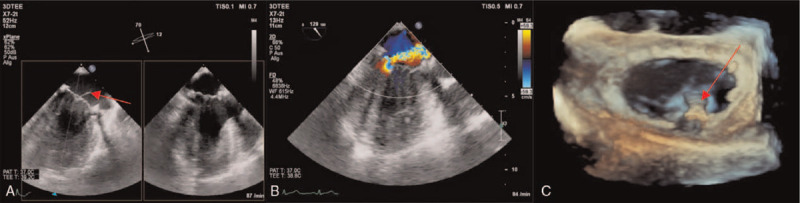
TEE of mitral valve after surgical repair with recurrent regurgitation before intervention. Panel A: Biplane view of the mitral valve. Red arrow indicates a flail leaflet. Panel B: Long axis view of the mitral valve with an anteriorly directed, eccentric regurgitation jet. Panel C: 3D surgeon's view of the mitral valve with annuloplasty ring. Red arrow points to the NeoChord device that is located posteriorly between segments P_2_ and P_3_ and passing through the valve from the left ventricle into the left atrium. 3D = three dimensional, TEE = transesophageal echocardiography.
